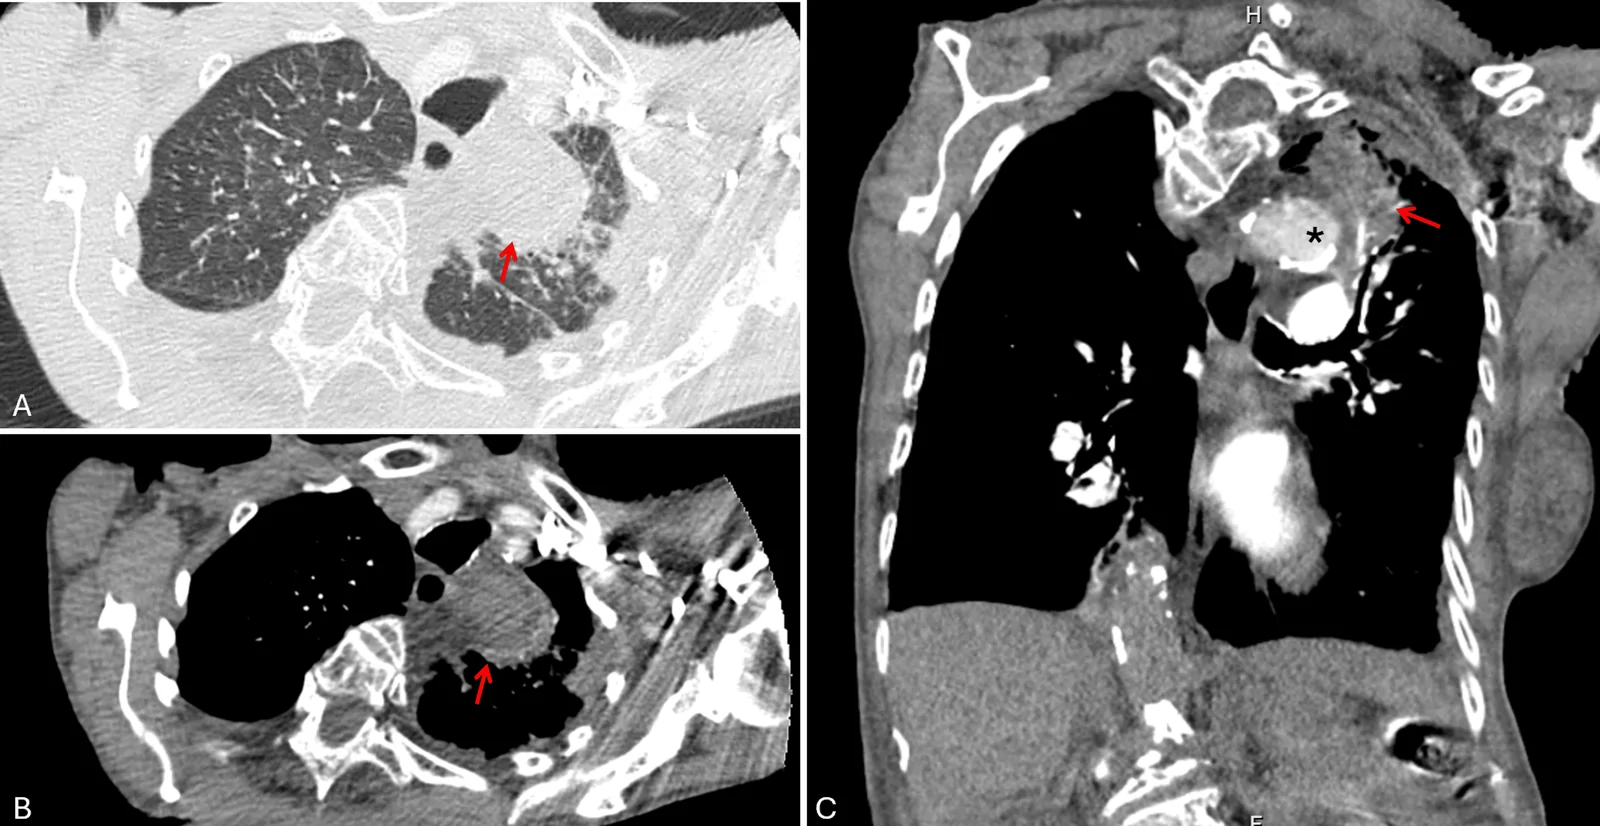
Chest CTA images obtained using a PE protocol. (A) Axial lung window. (B) Axial mediastinal window. (C) Coronal reformatted image. A soft-tissue density lesion (red arrow) is observed in close contact with the aortic arch (*), initially interpreted as a pulmonary mass. CTA: computed tomography angiography, PE: pulmonary embolism.
